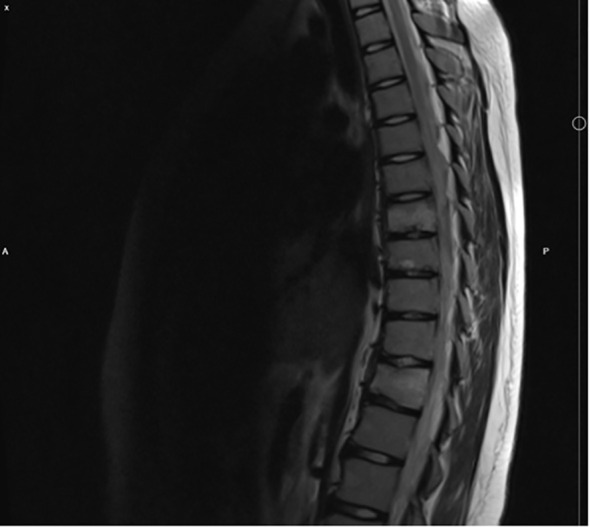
MRI of the spine, showing compression fractures of the Th7, Th8 and Th11.
